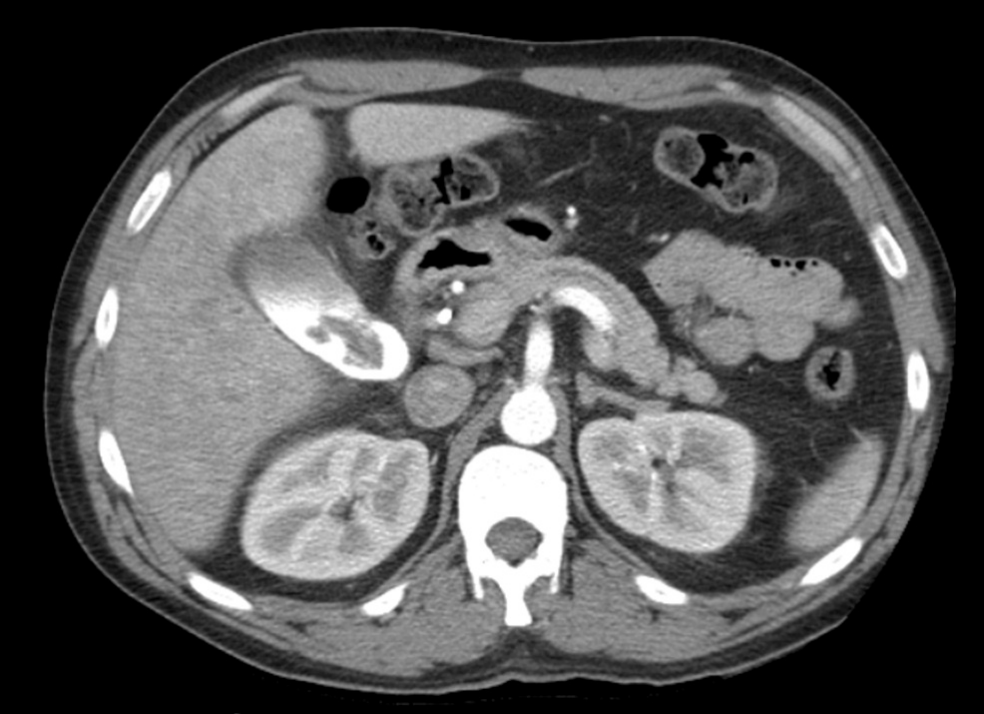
Axial view of CT angiography reveals an irregular filling defect in the gallbladder lumen, consistent with hemorrhage.
